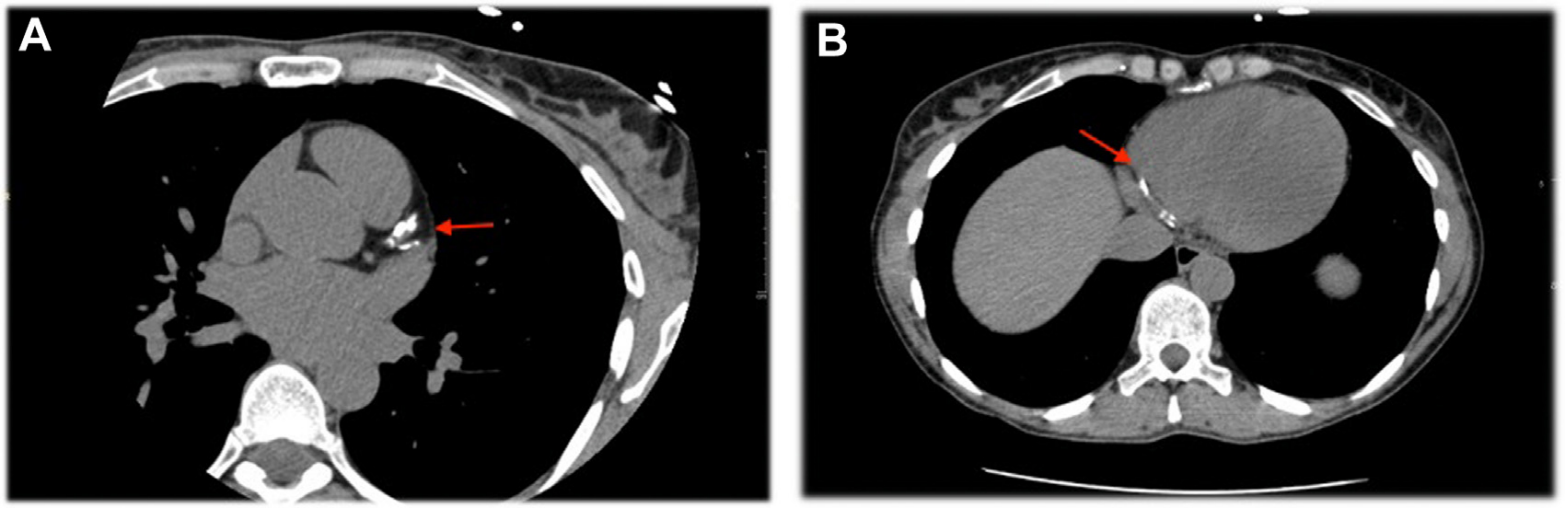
Coronary Artery Computed Tomography Coronary artery computed tomography demonstrating (A) coronary calcification of the left anterior descending artery and left circumflex artery (red arrow) and (B) coronary calcification of the right coronary artery (red arrow).
